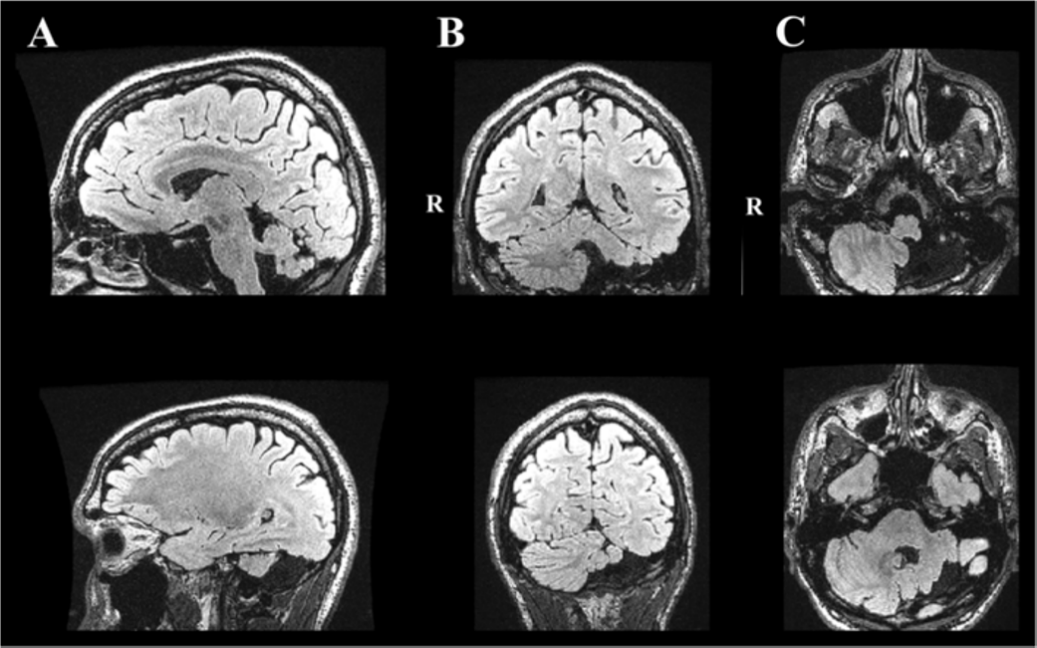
FLAIR-CUBE image of the encephalon showing left neocerebellar hypoplasia. A. Sagittal slices. B. Coronal slices. C. Axial slices passing through the posterior fossa. R. Right side.
